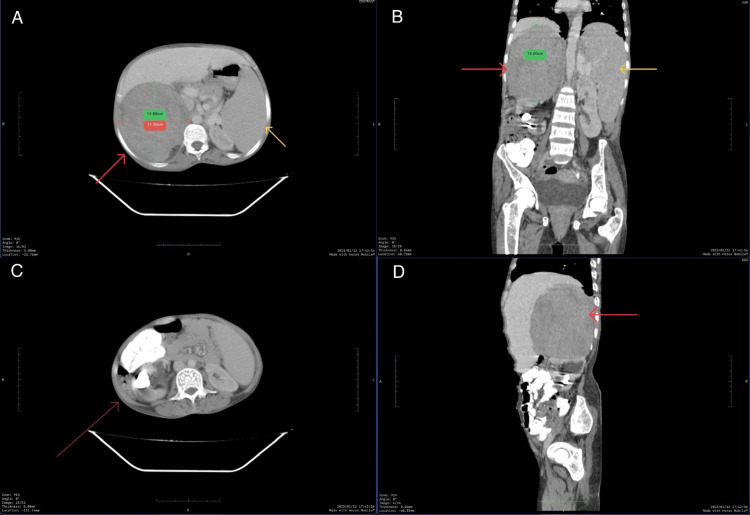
CT scan of abdomen and pelvis with contrast The images show (a) the axial section of the right suprarenal mass (red arrow) and splenomegaly (yellow arrow); (b) the coronal section of the right suprarenal mass (red arrow) and splenomegaly (yellow arrow); (c) axial section of right renal calculus (red arrow); and (d) sagittal section of right supra renal mass (red arrow) CT: computed tomography
